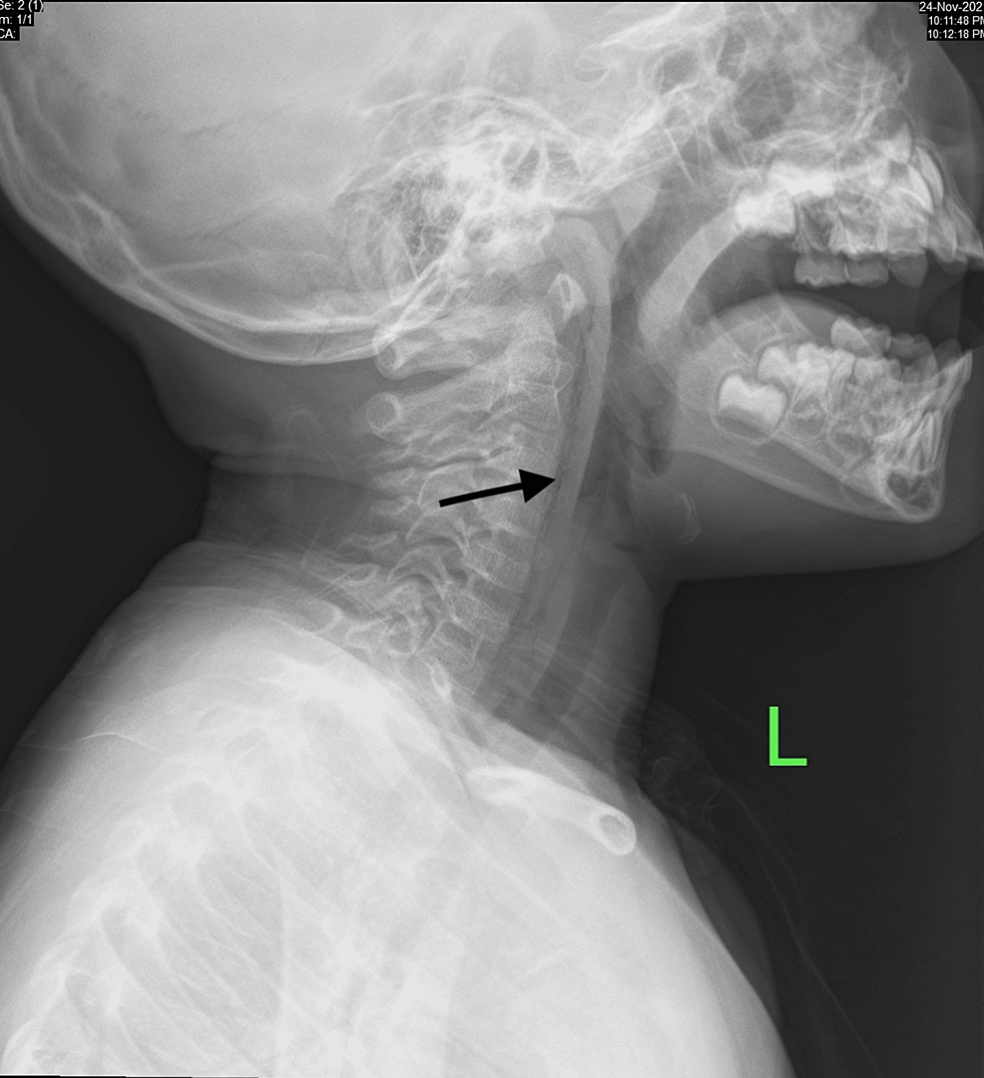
Lateral soft tissue radiography of the neck demonstrates a linear radiolucent line representing air in the retropharyngeal space
